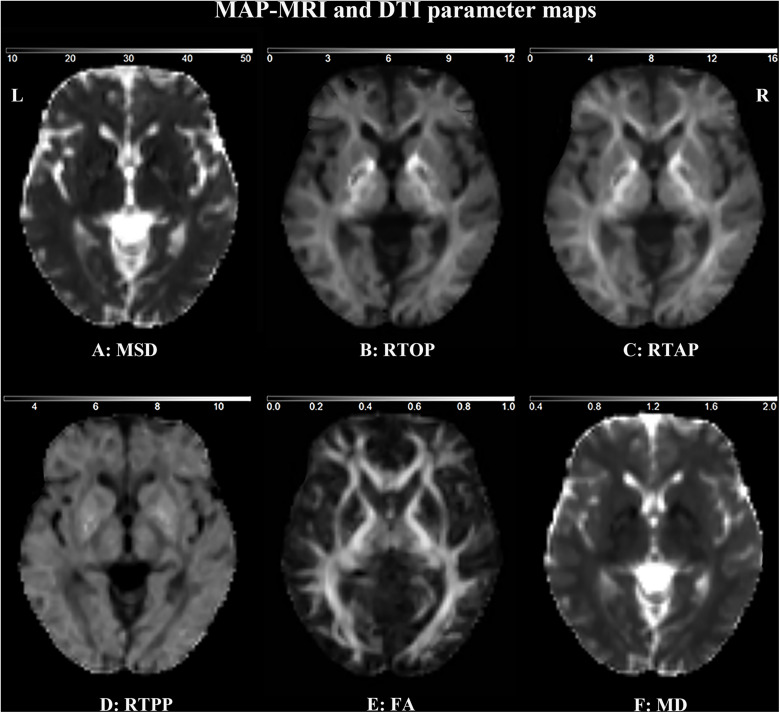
MAP MRI and DTI parameter maps of a representative healthy subject. MSD (A), RTOP (B), RTAP (C) and RTPP (D) are derived from MAP MRI, and FA (E), and MD (F) are derived from DTI. FA is dimensionless; MD, MSD, RTOP, RTAP, and RTPP are expressed in 10^–3^ mm^2^ s^–1^, 10^–5^ mm^2^, 10^5^ mm^–3^, 10^3^ mm^–2^, and 10^1^ mm^–1^, respectively.
